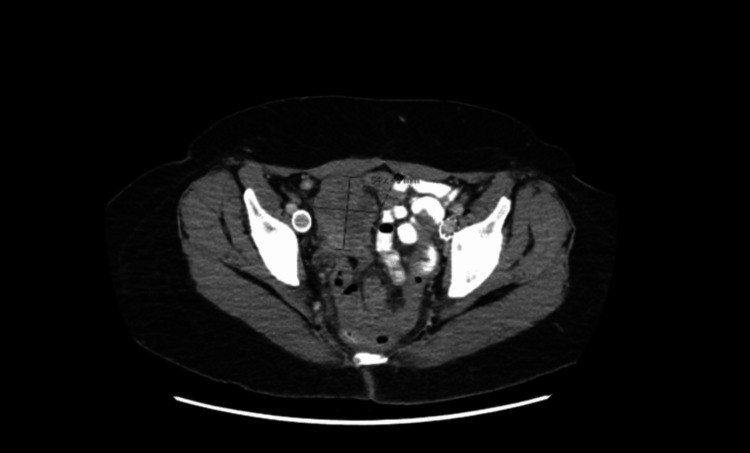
Axial slice CT demonstrating large abdominopelvic mass adjacent to bowel with the right ureteral compression causing hydronephrosis
